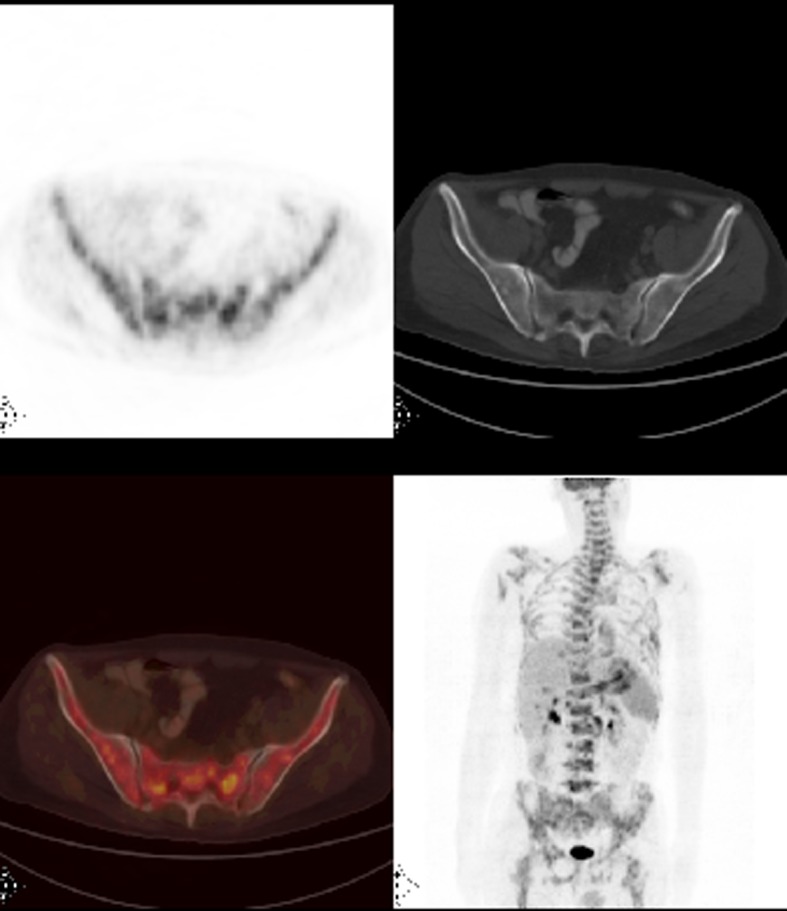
Axial PET/CT and MIP (maximum intensity projection) FDG PET/CT images revealed heterogeneously widespread increased FDG uptake at the skeleton which suggests bone marrow involvement.
